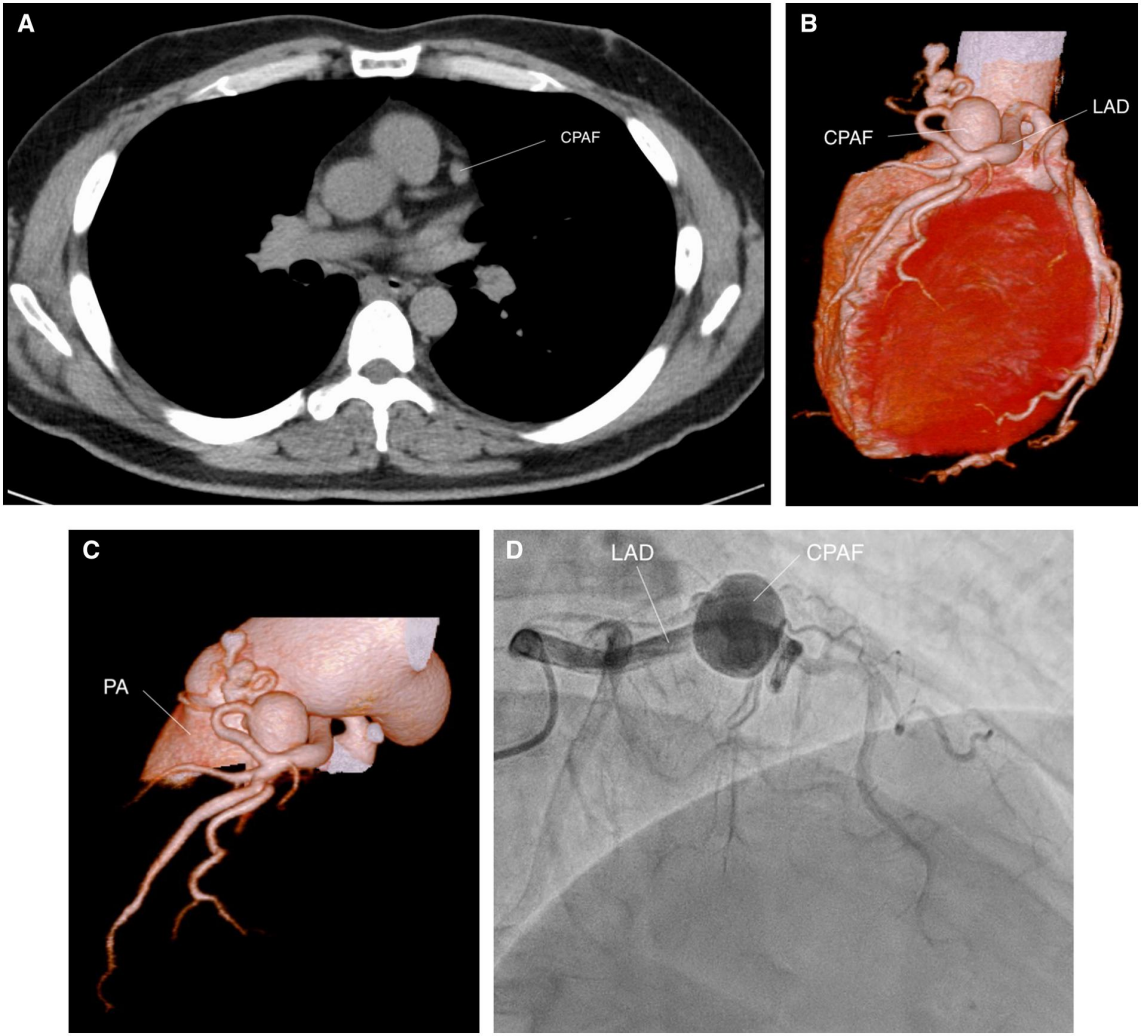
Preoperative images. (A) 3 years before, (B) 3D CTA, (C) CPAF and PA trunk and (D) CAG. CAG: coronary angiography; CPAF: Coronary-pulmonary artery fistula; CTA: computed tomography angiography; PA: pulmonary artery.
